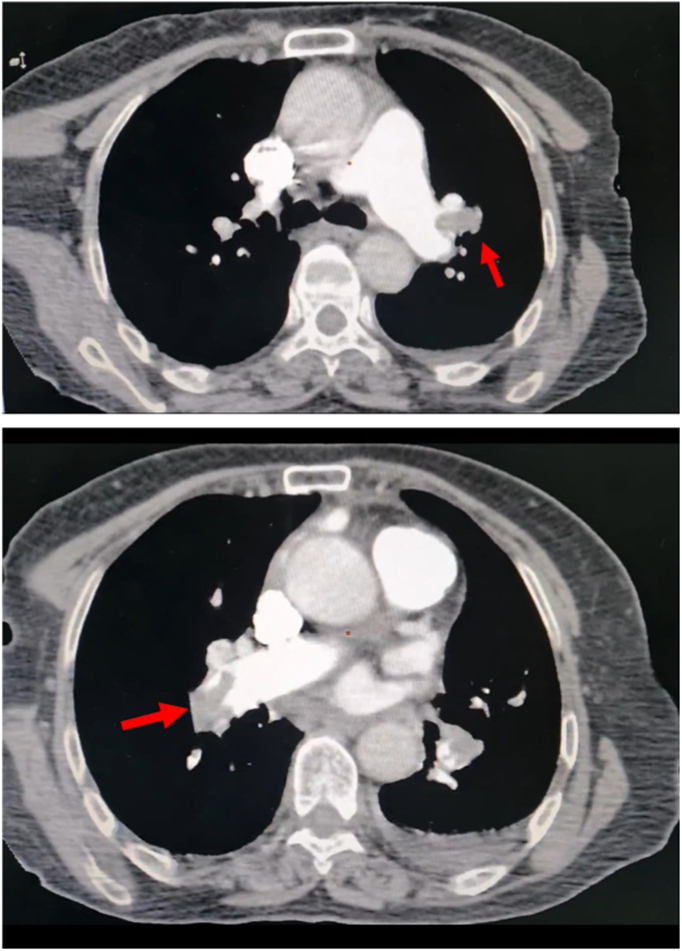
Chest CT scan with contrast injection showing hypodense material in both pulmonary arteries (red arrow) in favor of a pulmonary embolism. (For interpretation of the references to colour in this figure legend, the reader is referred to the Web version of this article.)
